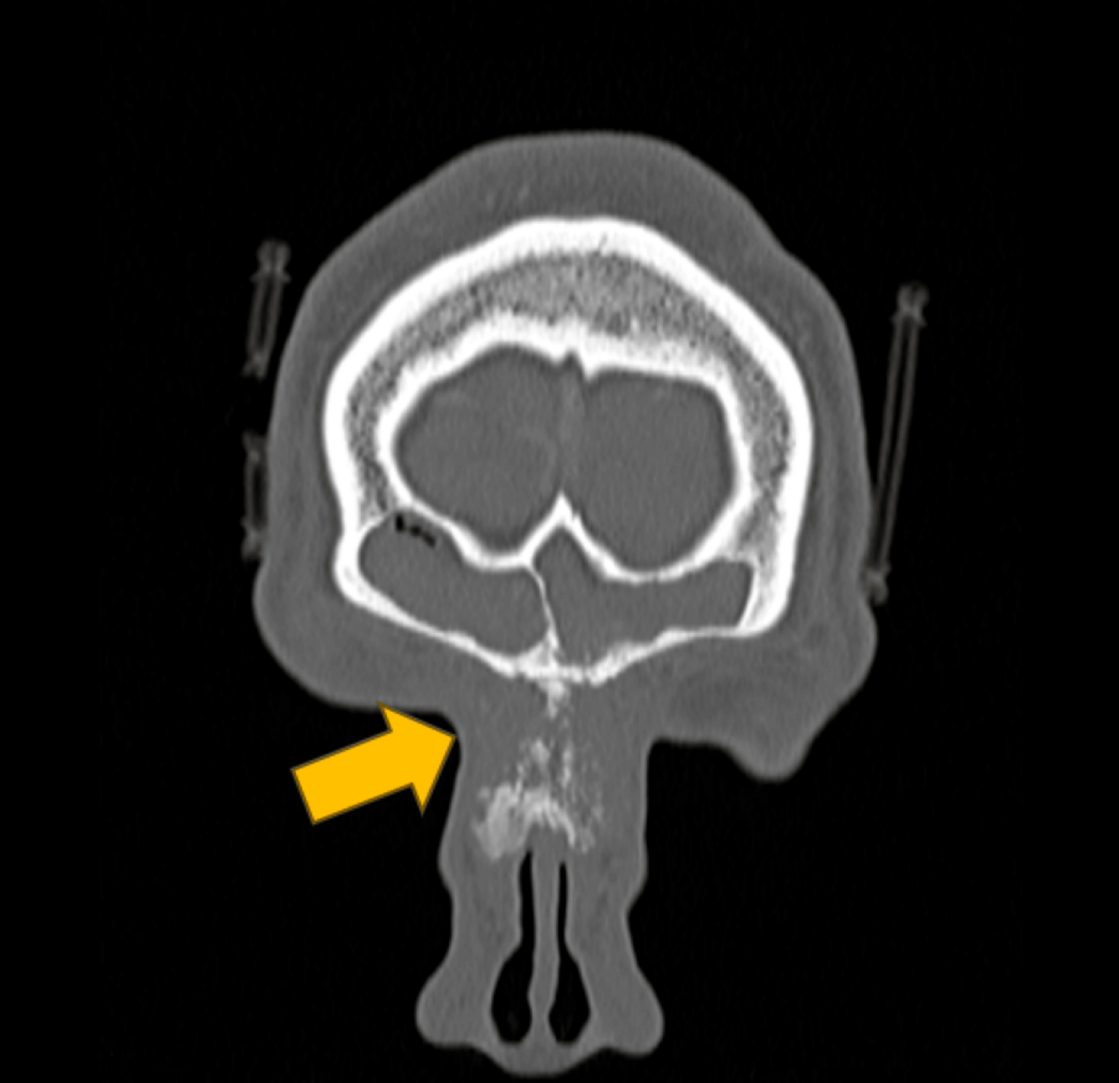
Homogenous enhancing soft tissue density within the left frontal sinus and aggressive sunburst periosteal reaction of nasal bone seen on CT scan (coronal plane, bone window) CT: computed tomography
